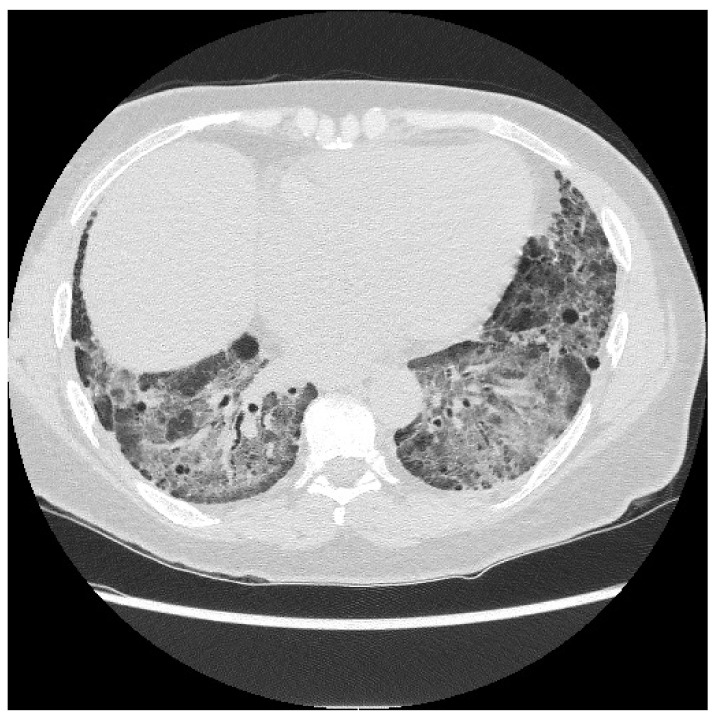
CT of the chest in the lower lung fields showing interstitial lung disease with fibrosis mostly in the lower lung fields.
